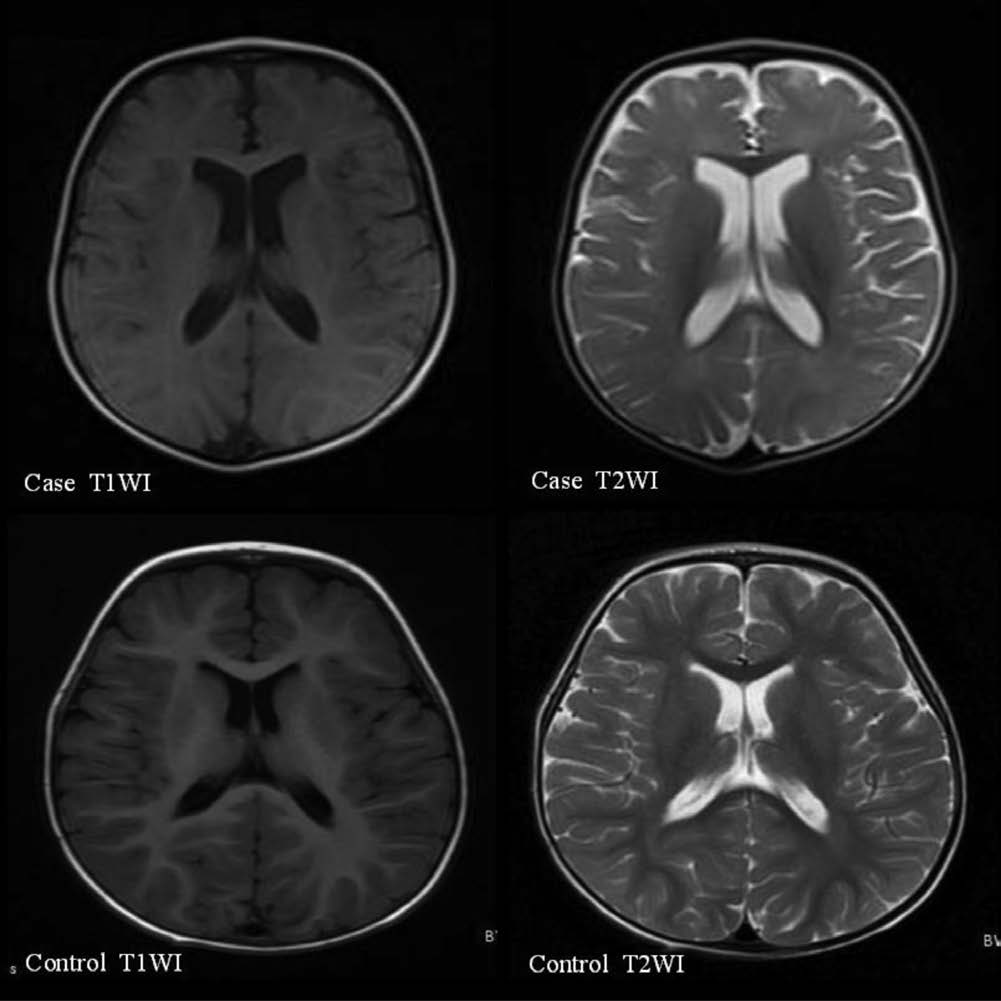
Brain MRI of the patient at 1 year and 5 months showed slightly delayed myelination in the brain and slight enlargement of bilateral lateral ventricles. MRI = magnetic resonance imaging.
